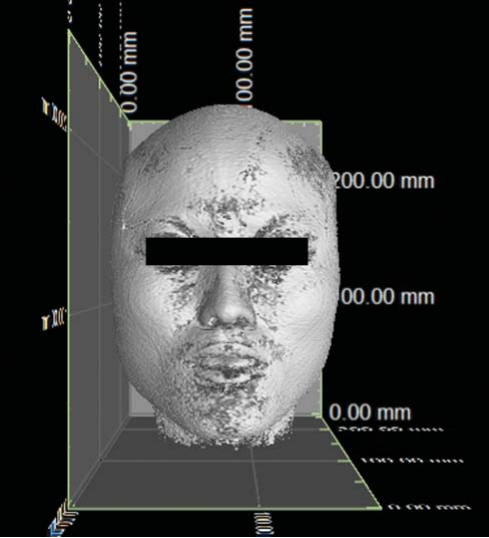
The stereogram of the three-dimensional reconstruction of MRI data.
